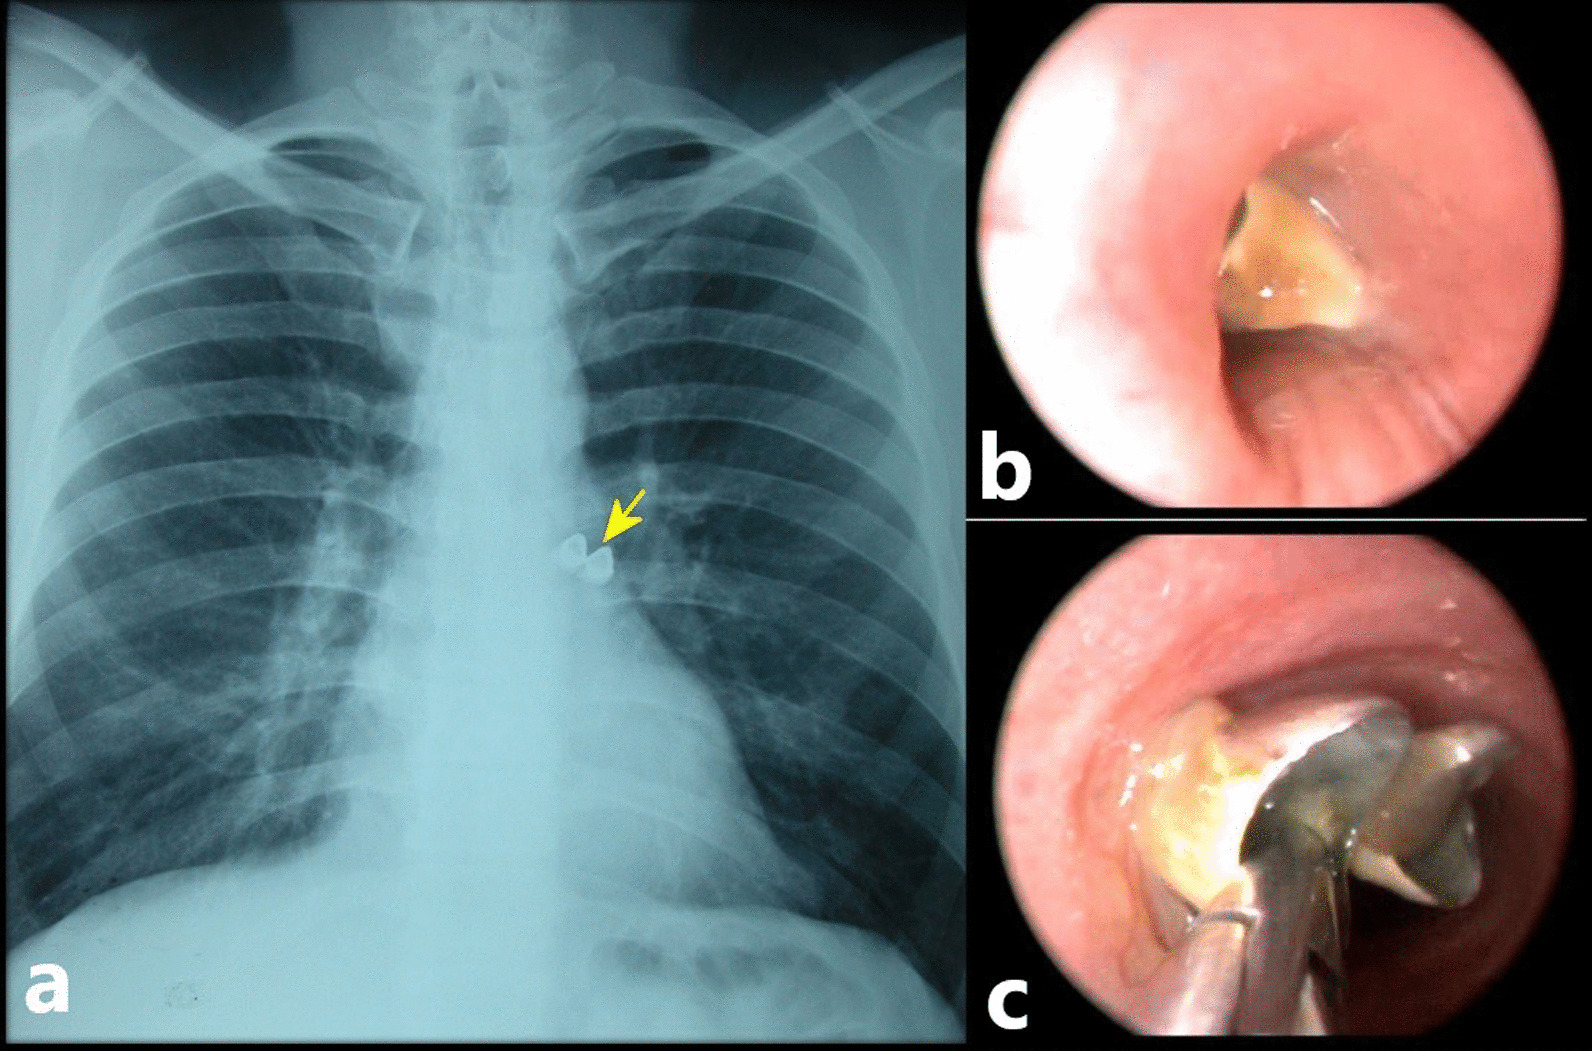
Removal of partial upper anterior tooth prosthesis with rigid bronchoscope. a Posterior-Anterior (PA) chest X-ray; black arrow shows foreign body in the left main bronchus. b, c Rigid bronchoscopy view of upper anterior tooth prosthesis
